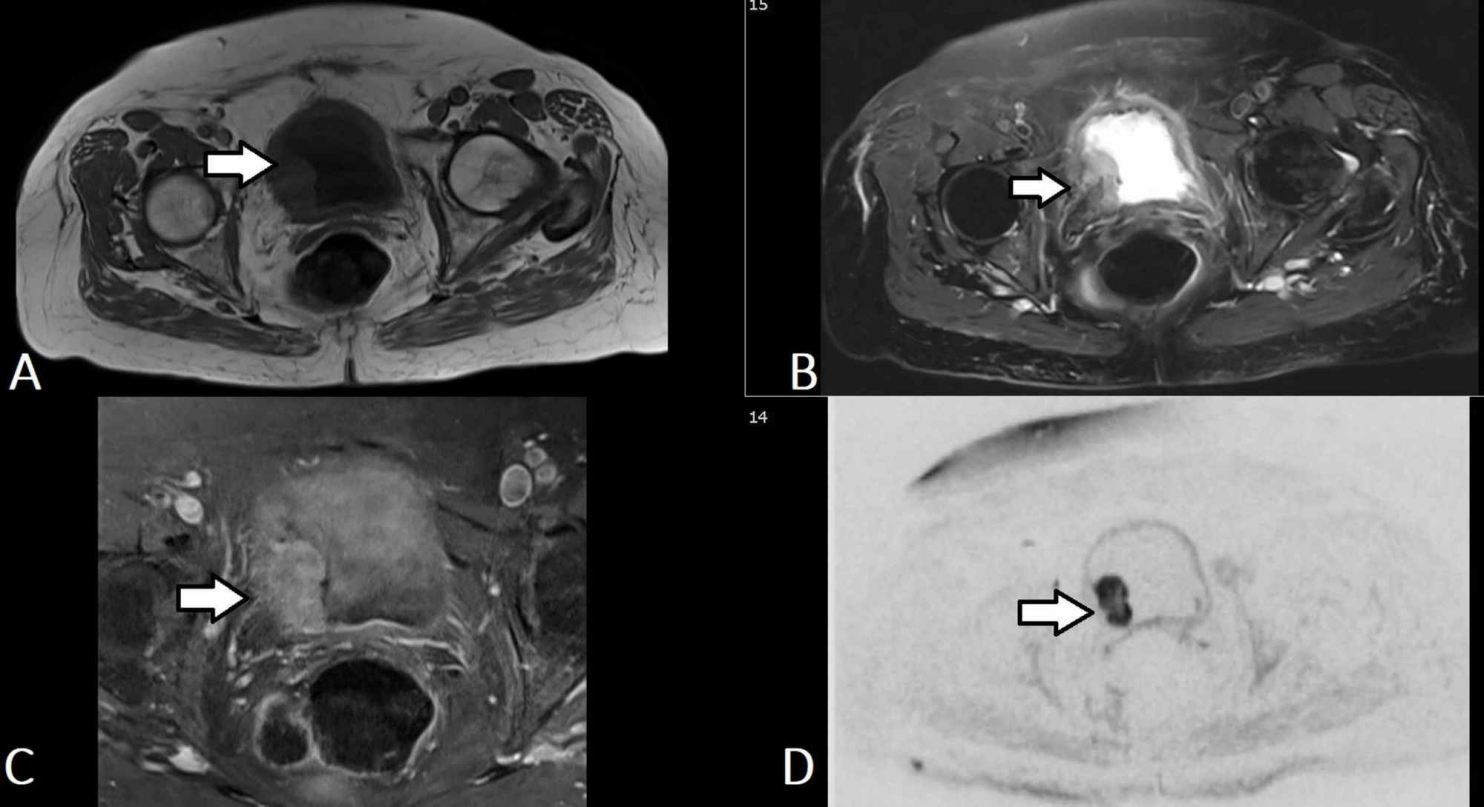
Bladder TCC (MRI) (A-D) Selected axial MR urography images of the same patient demonstrate a lobulated mass (arrow) arising from the right lateral wall of the urinary bladder which extends to the trigone and invades into the urinary bladder wall to involve the outer layer without extension into the perivesical fat. The mass is of T1 low and T2 high signal intensity. The mass demonstrates relatively homogeneous, diffuse contrast enhancement and prominent diffusion restriction (b50/400/800). The constellation of findings reflects a bladder tumour which is probably staged as T2b No Mx. TCC: Transitional cell carcinoma
